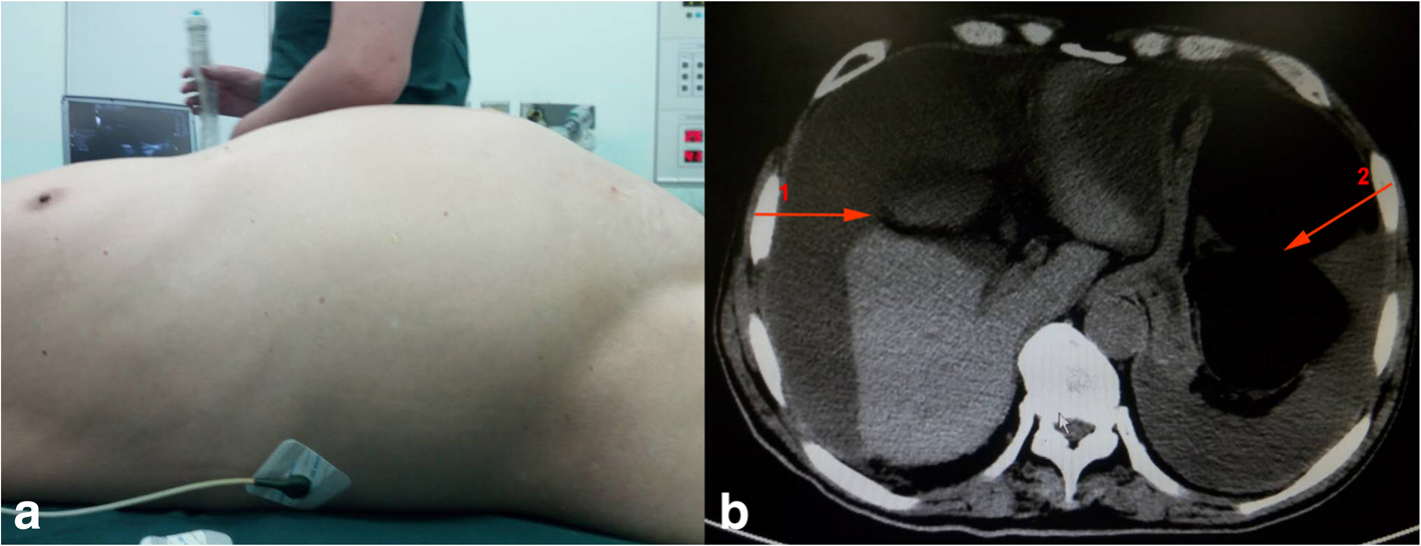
Physical examination and abdominal computed tomography scans. Physical examination revealed abdominal distension (a). Abdominal computed tomography images of the patient revealed peritoneal effusion (arrow 1) and bowel dilatation (arrow 2) (b)
